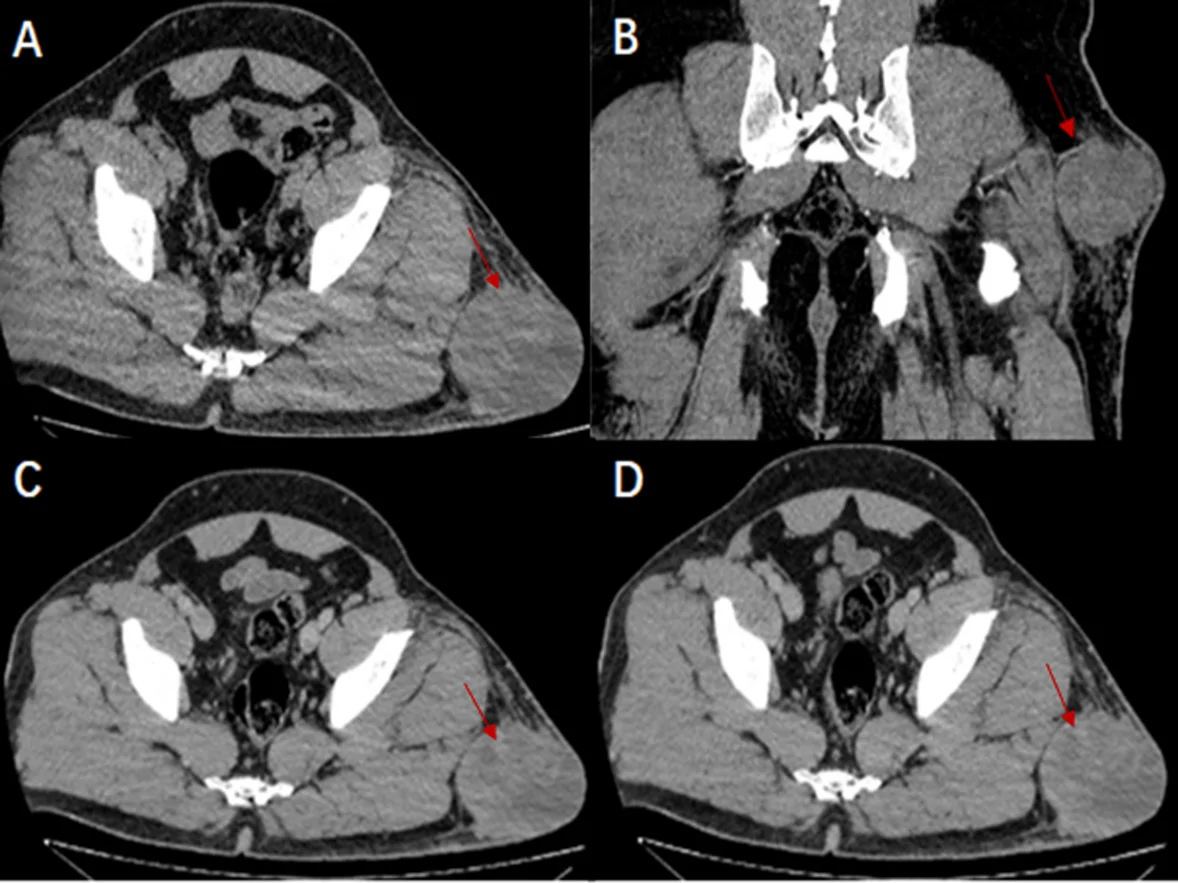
Contrast-enhanced CT of the pelvis demonstrating a large subcutaneous mass in the left gluteal region. A Axial non-contrast CT showing a well-defined soft tissue lesion. B Coronal reconstruction demonstrating the superficial location of the tumor (arrow) and its separation from the underlying bone. C Axial contrast-enhanced image showing heterogeneous enhancement. D Delayed-phase CT demonstrating mild to moderate delayed enhancement
